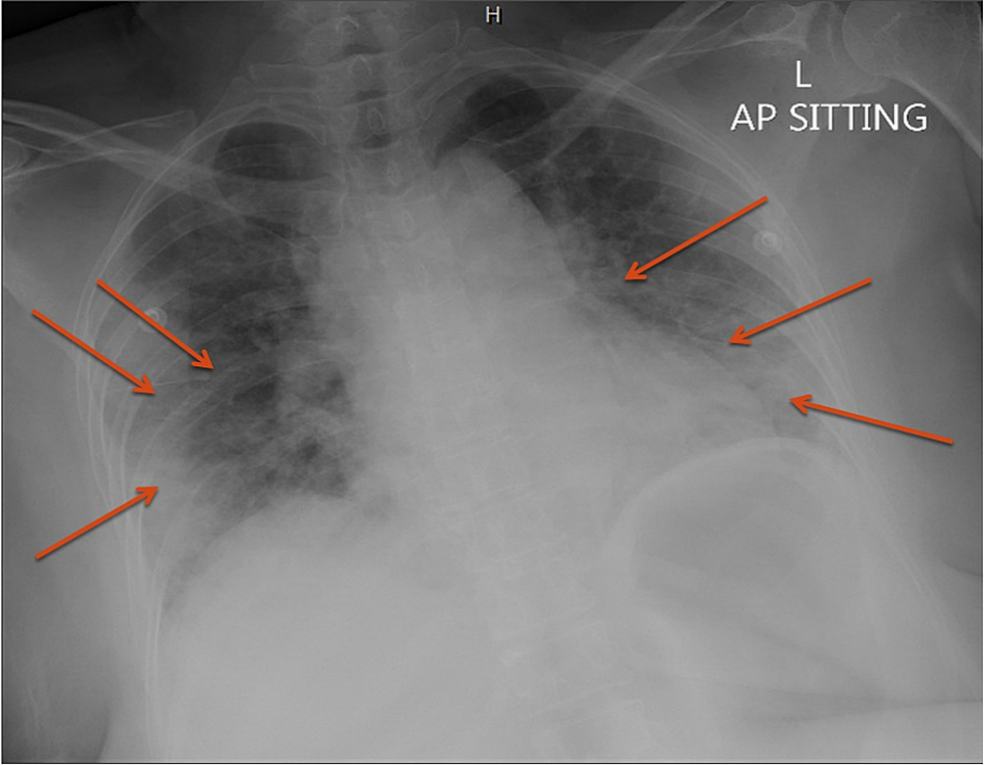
Chest x-ray showing bilateral pulmonary consolidations. AP: anteroposterior
